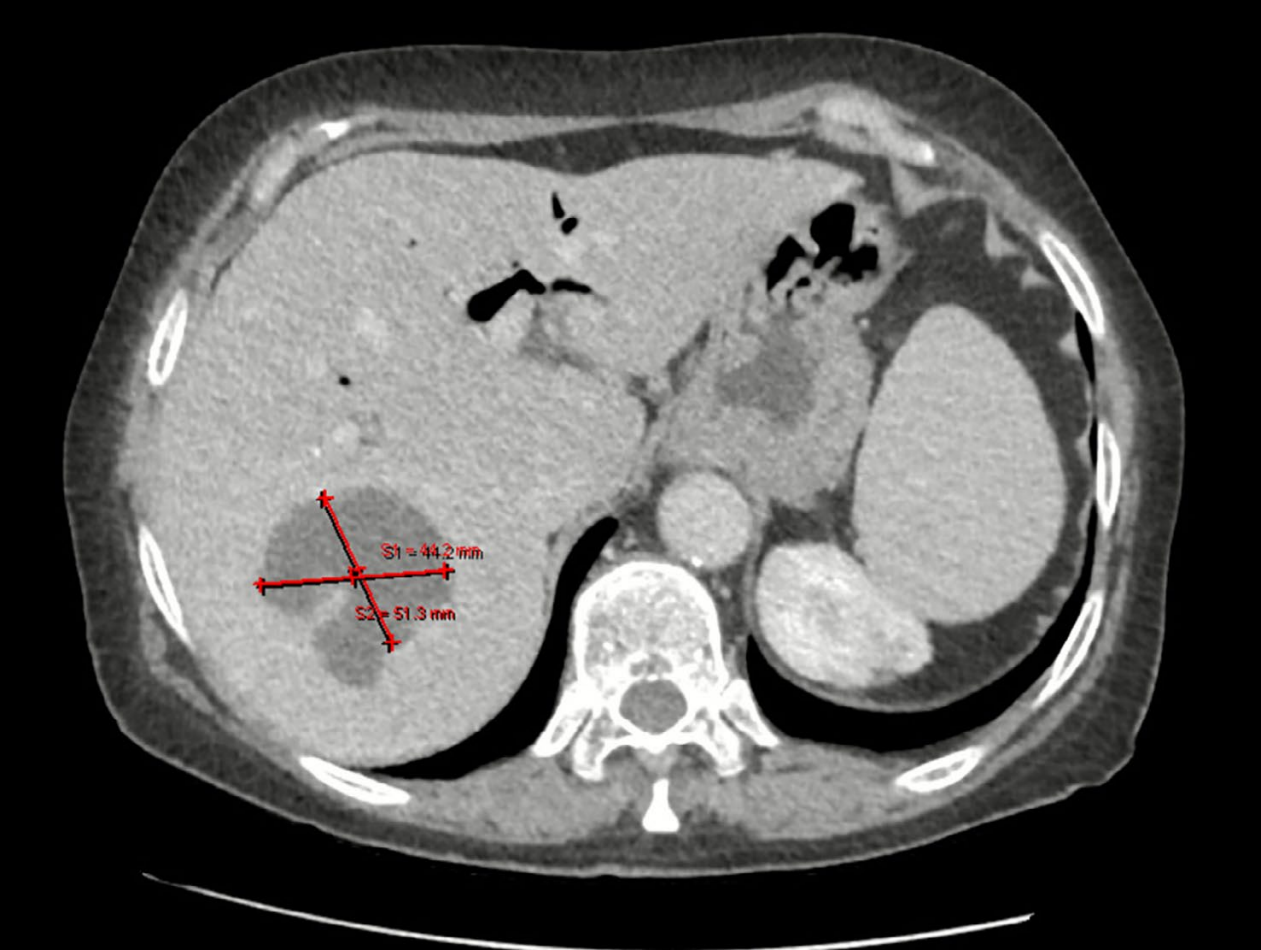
Contrast‐enhanced CT scan of the abdomen showing a pyogenic liver abscess in the right lobe.
